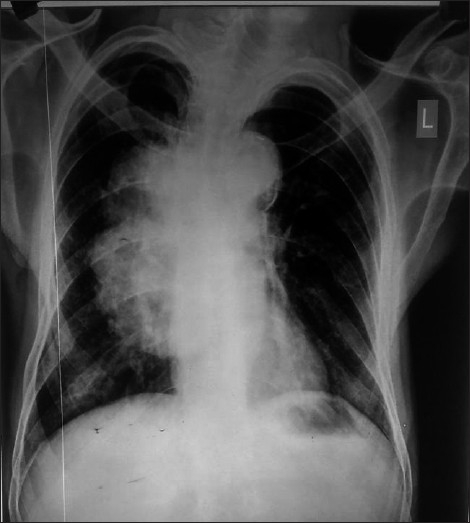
Chest X-ray showing right Hilar mass which was found malignant on bronchoscopy
